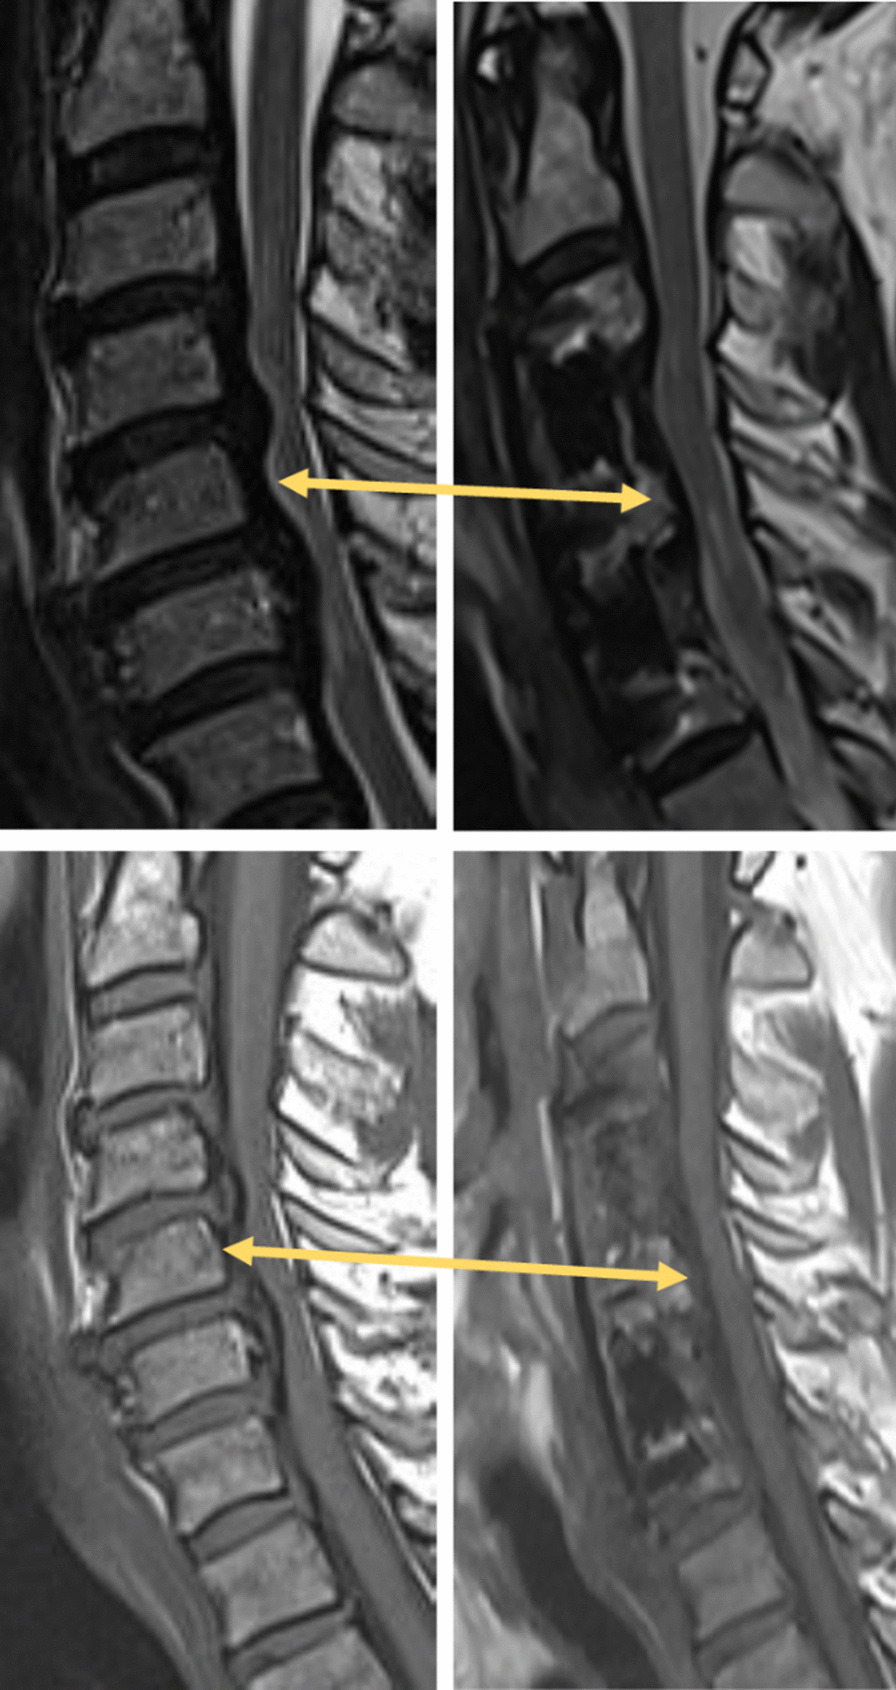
MRI scans of a patient treated for C4-C7 stenosis. Top: T2. Bottom: T1. Left: preoperative scan. Right: postoperative scans, showing the implant and the areas treated using the device. The arrows mark the area posterior to the preserved vertebra demonstrating the removal of cord compressive tissue by the Dreal. Note the diameter of the cord in the T1 images pre- and post-surgery
